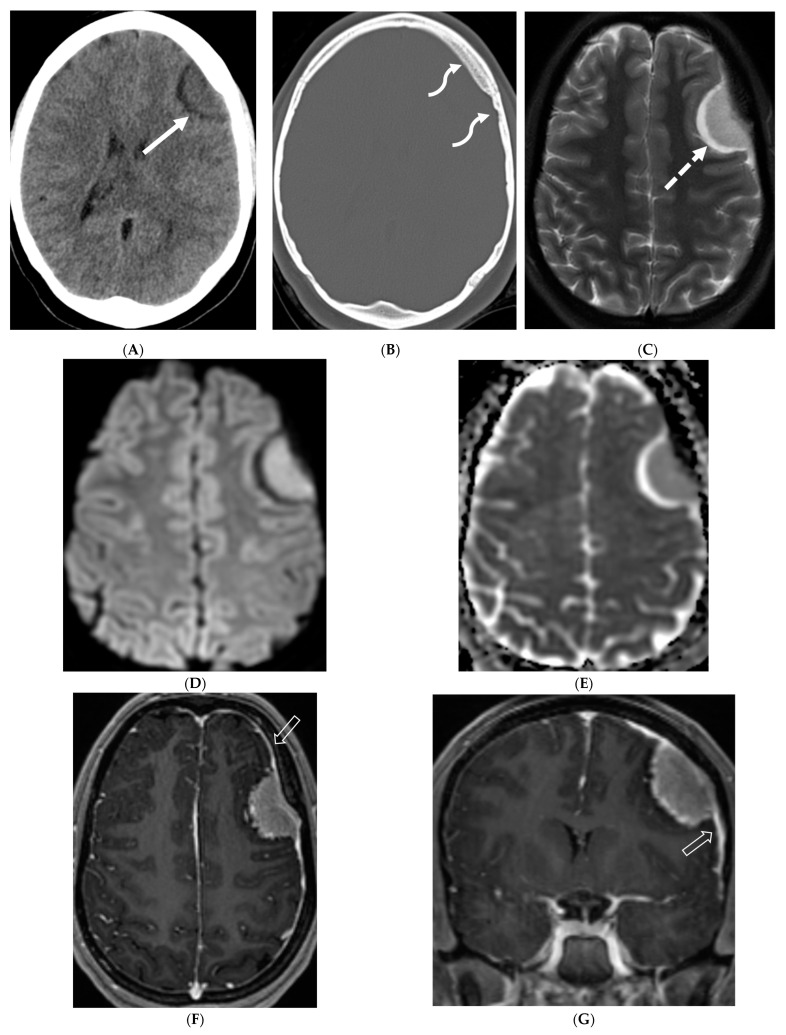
Axial CT (A,B), axial T2 fat saturated (FS) (C), Axial Diffusion Weighted Imaging (DWI) & Apparent Diffusion Coefficient (ADC) (D,E), axial and coronal post contrast (F,G): 15-year-old girl with nausea and headaches. CT shows iso-dense dural-based mass in the left anterior cranial fossa (arrow). Adjacent bone is hyperostotic and has irregular cortex (curved arrow). The lesion is isointense with cortex, which is buckled inwards from the mass. A hyperintense rim surrounds the mass representing CSF cleft (dashed arrow). No significant restricted diffusion is noted. The mass enhances intensely and uniformly. A dural tail (open arrow) of benign, nonneoplastic reactive thickening is present adjacent to the left frontal mass, characteristic of classic “typical” WHO grade 1 meningioma.
